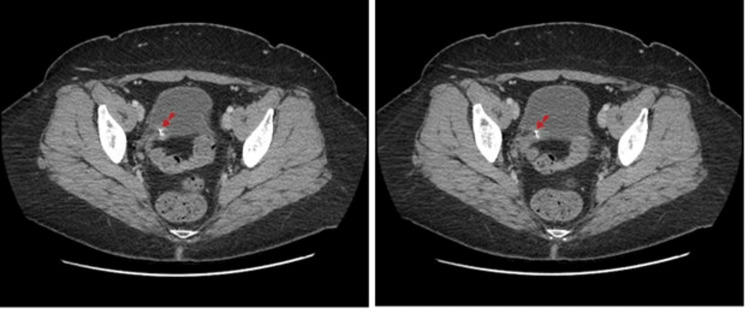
Computed tomography with contrast, axial plane. Device catheter (red dotted arrows) penetrating the vaginal cuff.
